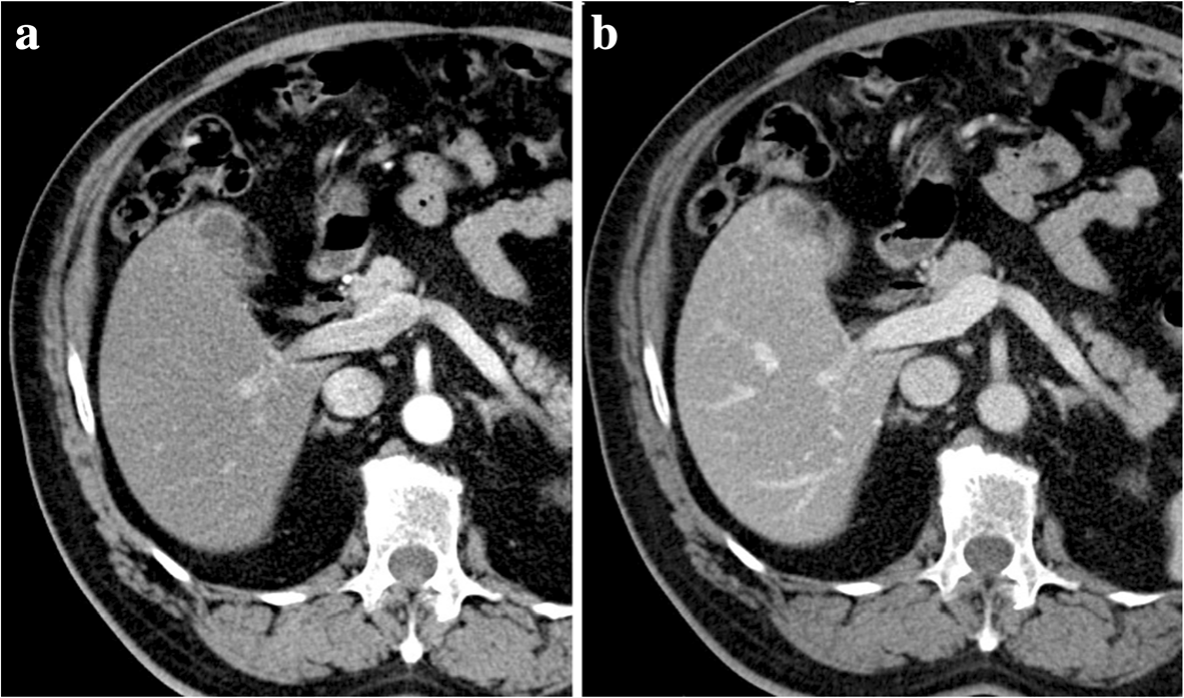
Pseudothrombosis phenomena. a Late arterial phase CT axial image shows a heterogeneous low-attenuation PV content, due to pseudothrombosis phenomena. b Accordingly, on portal venous phase, the PV shows homogeneous fulfilling, discarding a hypothesis of a true thrombosis
